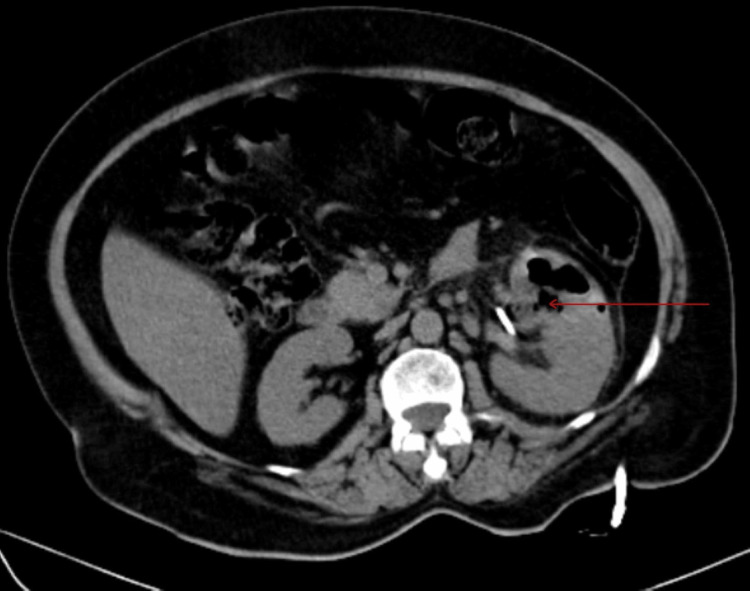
Non-contrast computed tomography of the abdomen showing reduction in air pockets within the left renal parenchyma after six days of initial scan.
